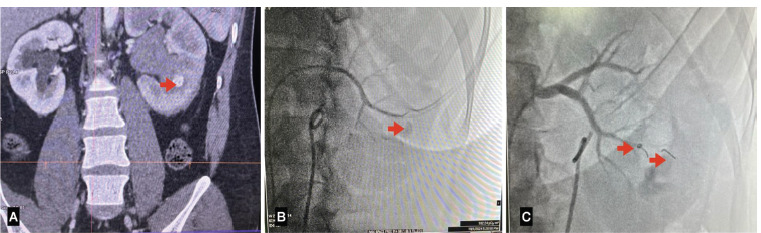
(A) CT Angiography image showing pseudo aneurysm in lower segmental left renal artery. (B) Diagnostic angiography showing two pseudoaneurysm in lower segmental renal artery of left kidney. (C) Post embolisation showing two coils in situ leading to occlusion of both pseudo aneyrysm.
